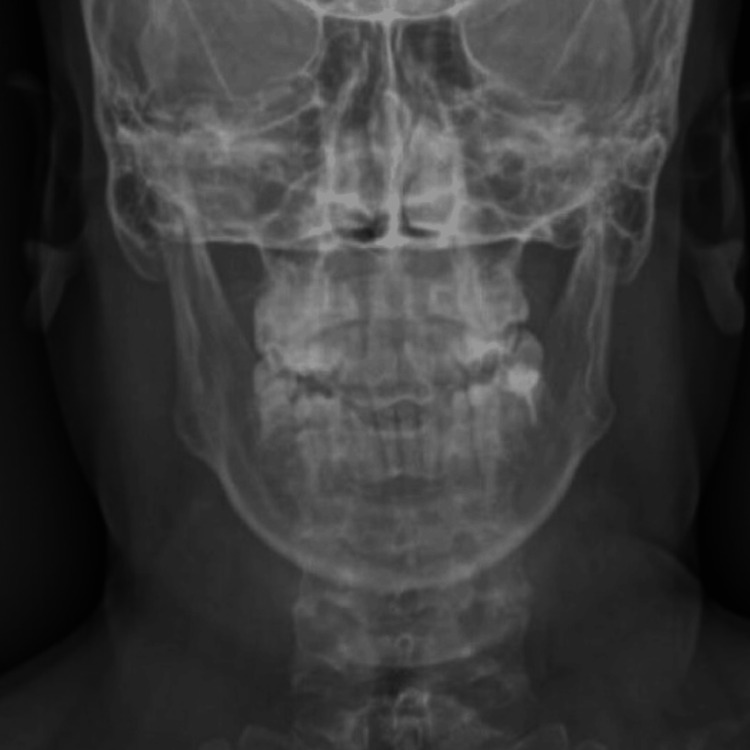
Frontal skull radiograph showing maintained cranial symmetry without features of sclerosing cranial dysplasia.
